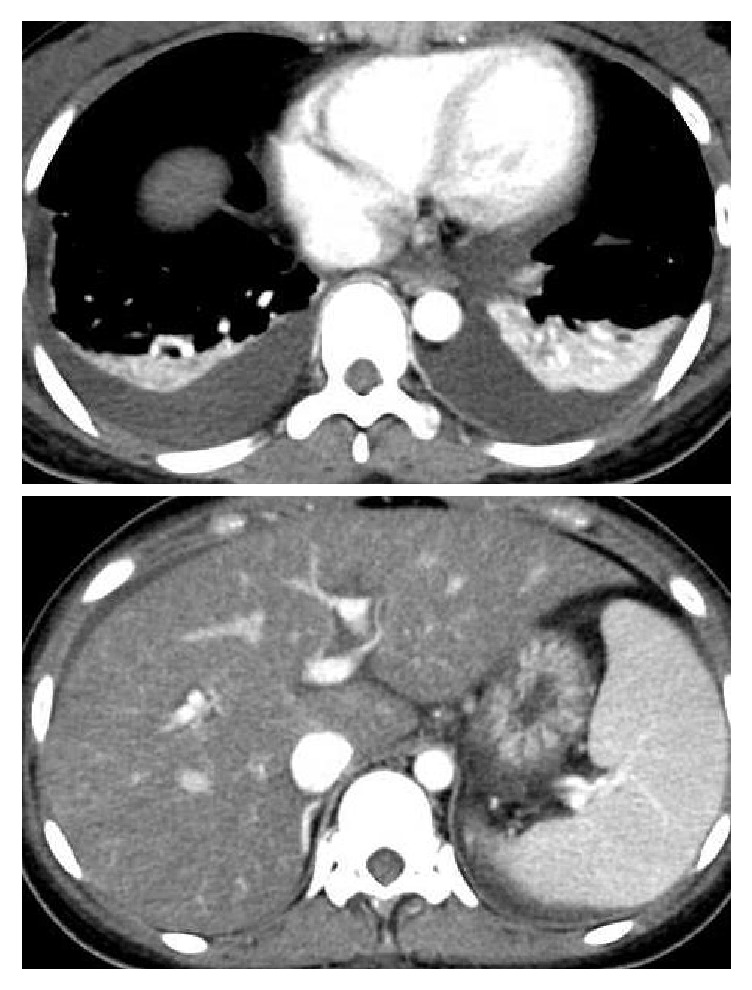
Dynamic contrast-enhanced CT scan.
